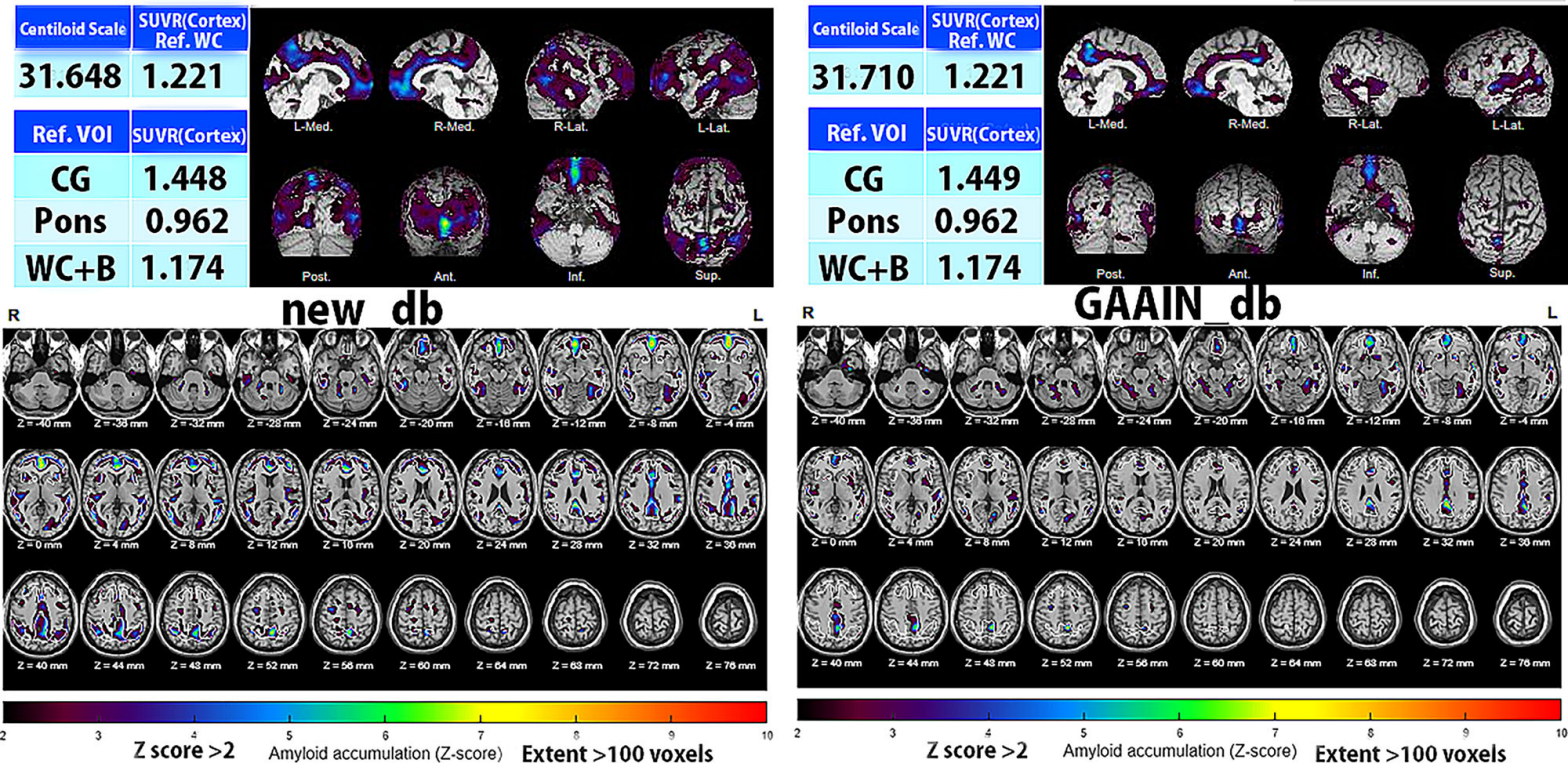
Comparison of analytical results by AMYclz between the new database and Global Alzheimer's Association Interactive Network (GAAIN) database. A *Z*‐score map is displayed by overlay on tomographic sections with a contour of the target cortical volume of interest (VOI) and with a surface rendering of the standardized brain MRI. A regionally higher *Z*‐score was obtained from the present new database (left) than from the GAAIN database (right).
